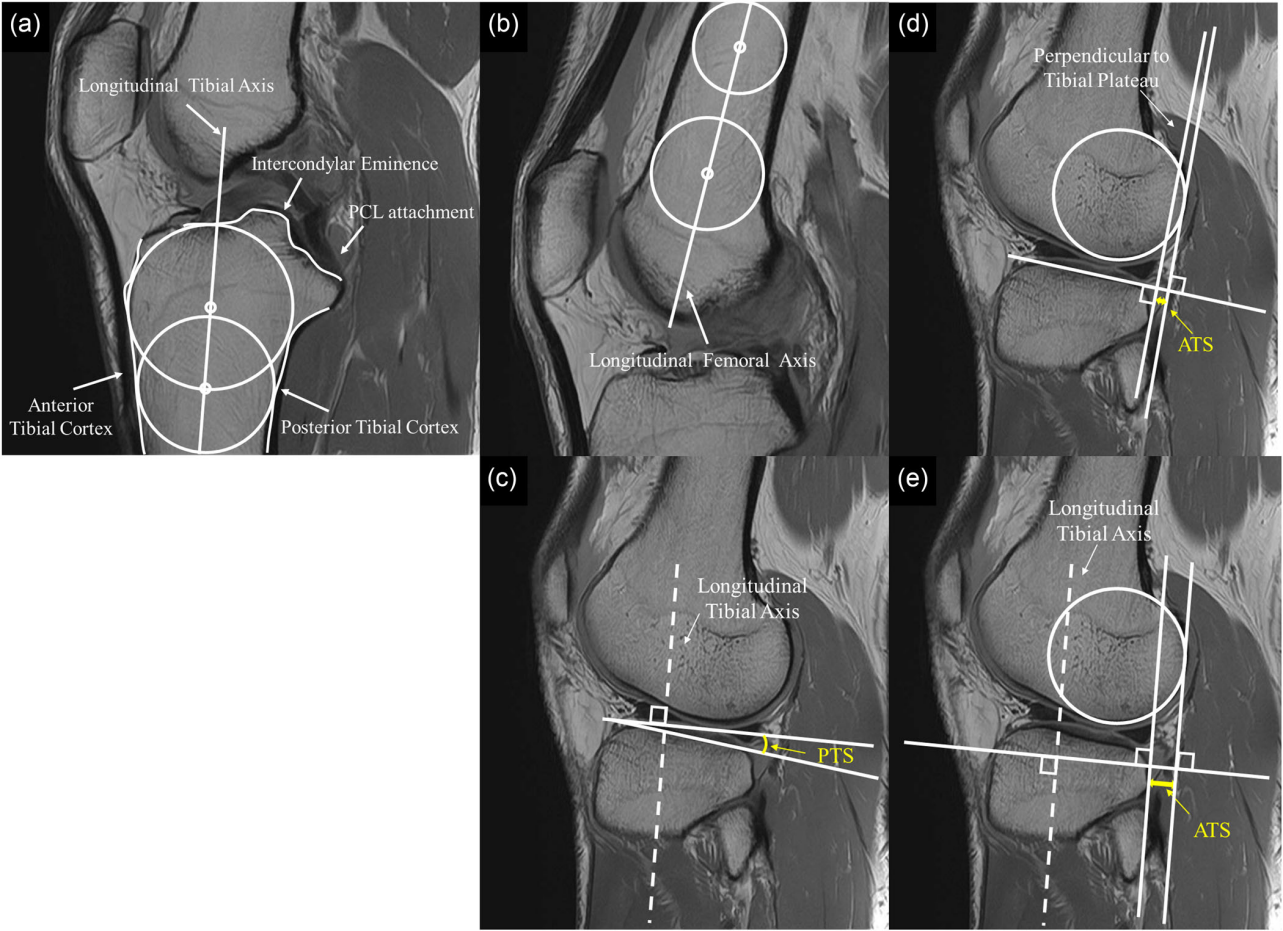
Measurements of knee angle, ATS and PTS on sagittal magnetic resonance imaging. (a) To define the longitudinal tibial axis, two circles were drawn. The tibial axis was defined as the line connecting the centres of these two circles. (b) To define the longitudinal femoral axis, two circles were drawn. The femoral axis was defined as the line connecting the centres of these two circles. (c) PTS was defined as the angle between the tibial plateau and a line perpendicular to the longitudinal tibial axis. (d) ATS measured with the plateau method was defined as the distance between a vertical line of tibial plateau drawn from the posterior margin to the posterior femoral condyle and the posterior edge of the tibial articular surface. (e) ATS measured with the bone axis method was defined as the distance between a parallel line of the tibial axis drawn from the posterior margin to the posterior femoral condyle and a parallel line drawn from the posterior margin of the tibial articular surface. ATS, anterior tibial subluxation; PCL, posterior cruciate ligament; PTS, posterior tibial slope.
